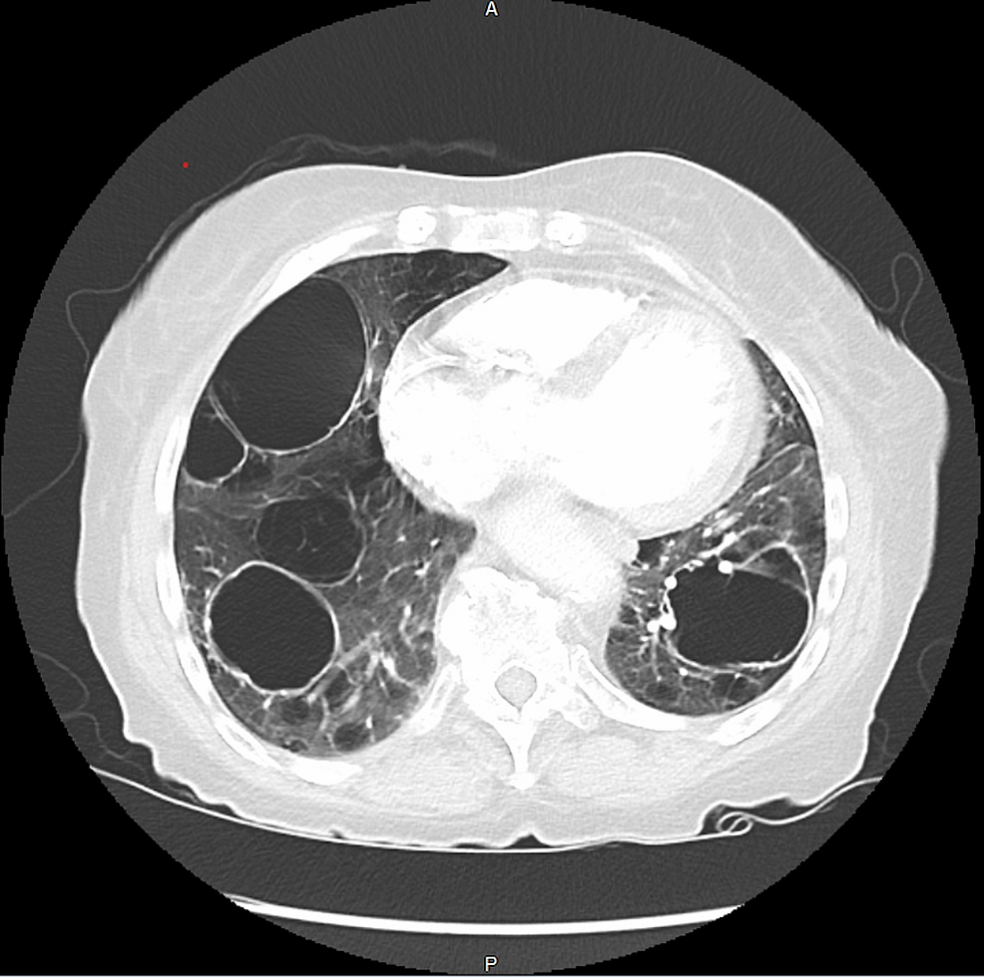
Axial view of the chest CT demonstrating multiple lung cysts
